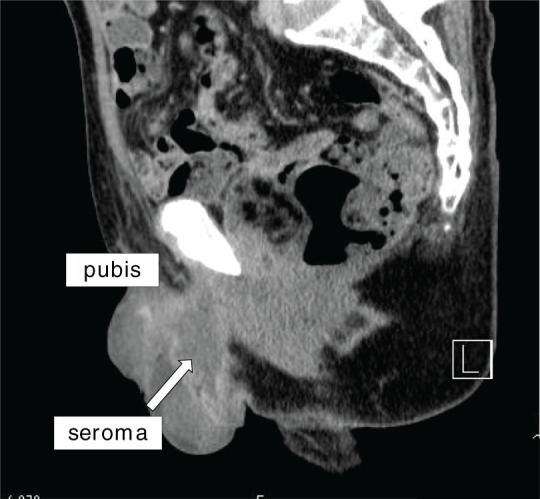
Postoperative contrast-enhanced CT of Case 1 at 3 months. The muscle flap demonstrates excellent blood supply (enhancement), and there is no evidence of hernia recurrence.
